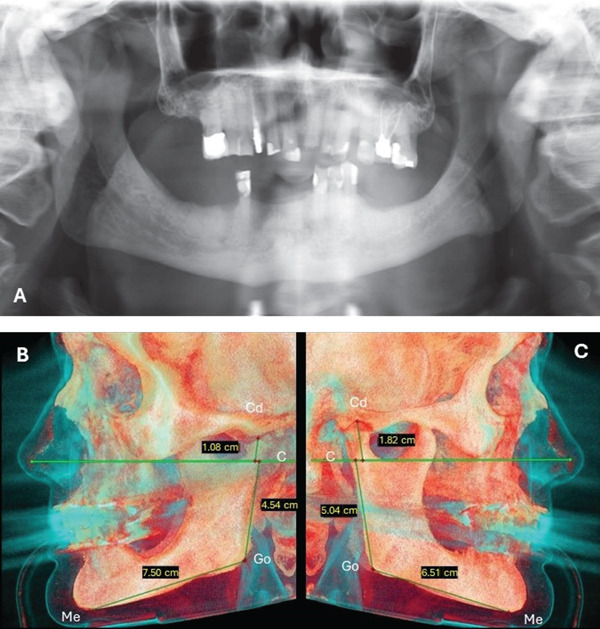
(A) Panoramic radiograph before starting dental treatment. (B, C): Left and right 3D CBCT images with linear measurements, indicating mandibular asymmetry. The following landmarks were used to measure the length of the condyle, ramus, and the body of the mandible: Cd: superior surface of the condyle, C‐point: lowest point of the semilunar incisure, Go: gonion, Me: menton.
